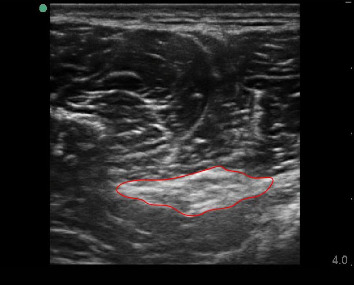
Ultrasound measurement of the cross-sectional area of the sciatic nerve. The high-echo imaging within the red irregular line indicates the cross-sectional area.
